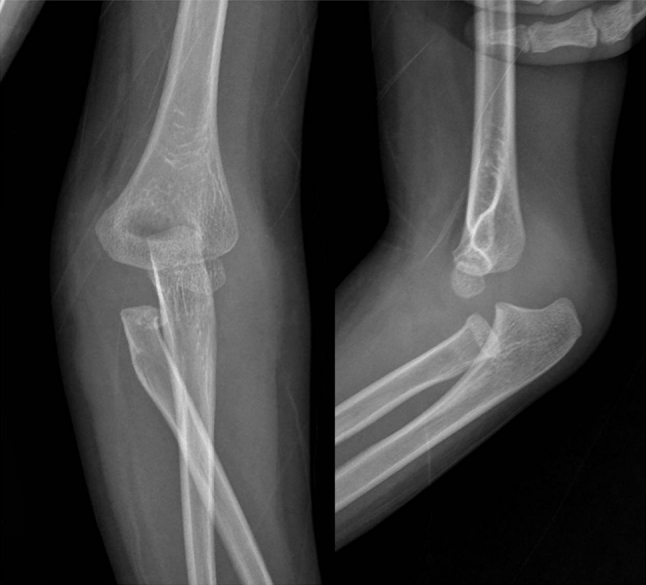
Initial anteroposterior and lateral radiographs show elbow dislocation and translocation of proximal radioulnar joint with radial neck fracture
